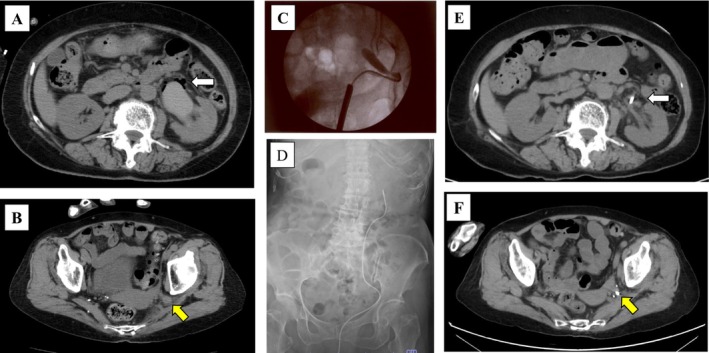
Radiographic images during the perioperative period for ureteral stenting. (A, B) Axial CT images of the emphysematous changes in the left pelvis (white arrow) and left ureter (yellow arrow). (C) Intraoperative fluoroscopic image of the left USH. (D) Plain abdominal radiograph after left ureteral catheter insertion. (E, F) Axial CT images at 4 days after ureteral catheter insertion. White arrow indicates left pelvis, and yellow arrow indicates left ureter.
